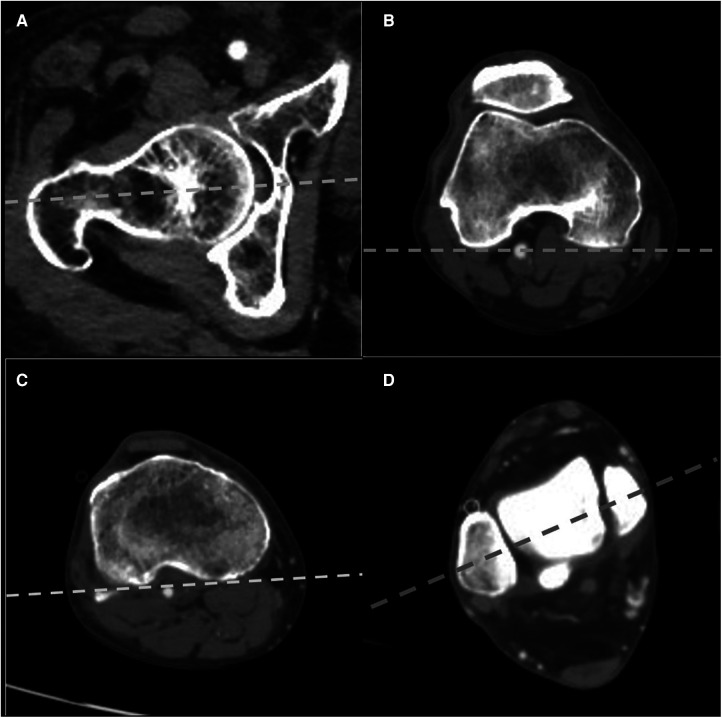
Measurement technique of lower limb rotation on CT (right limb). (A) Femoral neck axis. (B) Posterior condylar axis of the distal femur. (C) Axis of the proximal tibial condyles. (D) The bimalleolar axis. The data were recorded as an angle between the axis and horizontal baseline. CT, computed tomography.
